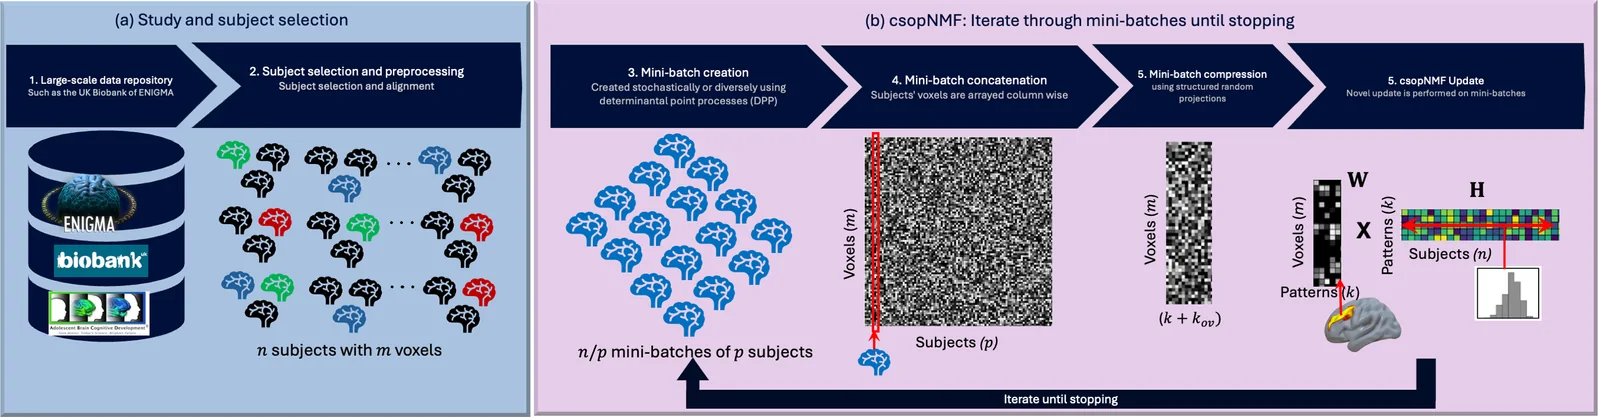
Overview of the proposed approach. (a) (1) A large-scale neuroimaging study of interest is selected for analysis. (2) Subjects meeting the inclusion criteria are identified, and their T1-weighted (T1w) images are processed using standard neuroimaging tools. (b) (3) Mini-batches are selected from the full sample either stochastically or using determinantal point processes (DPP) to promote diversity. (4) Images within each batch are vectorized and arrayed column wise to form mini-batch data matrices. (5) Mini-batches are compressed using structured random projections. (6) csopNMF is performed on compressed mini-batches, and model updates are accumulated across batches.
